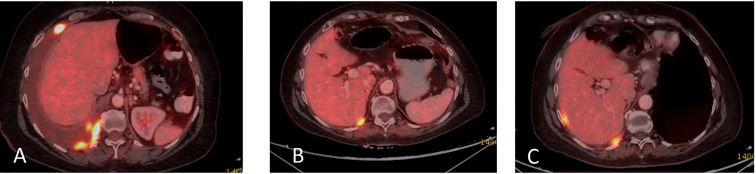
(A) Coronal PET-CT scan from November 2019 shows a pleural effusion and lesions with increased uptake of FDG-18, indicating disease progression. (B) Three months later, following Osimertinib demonstrated partial response. (C) February 2021, showing a new pleural lesion indicating disease progression on Osimertinib.
